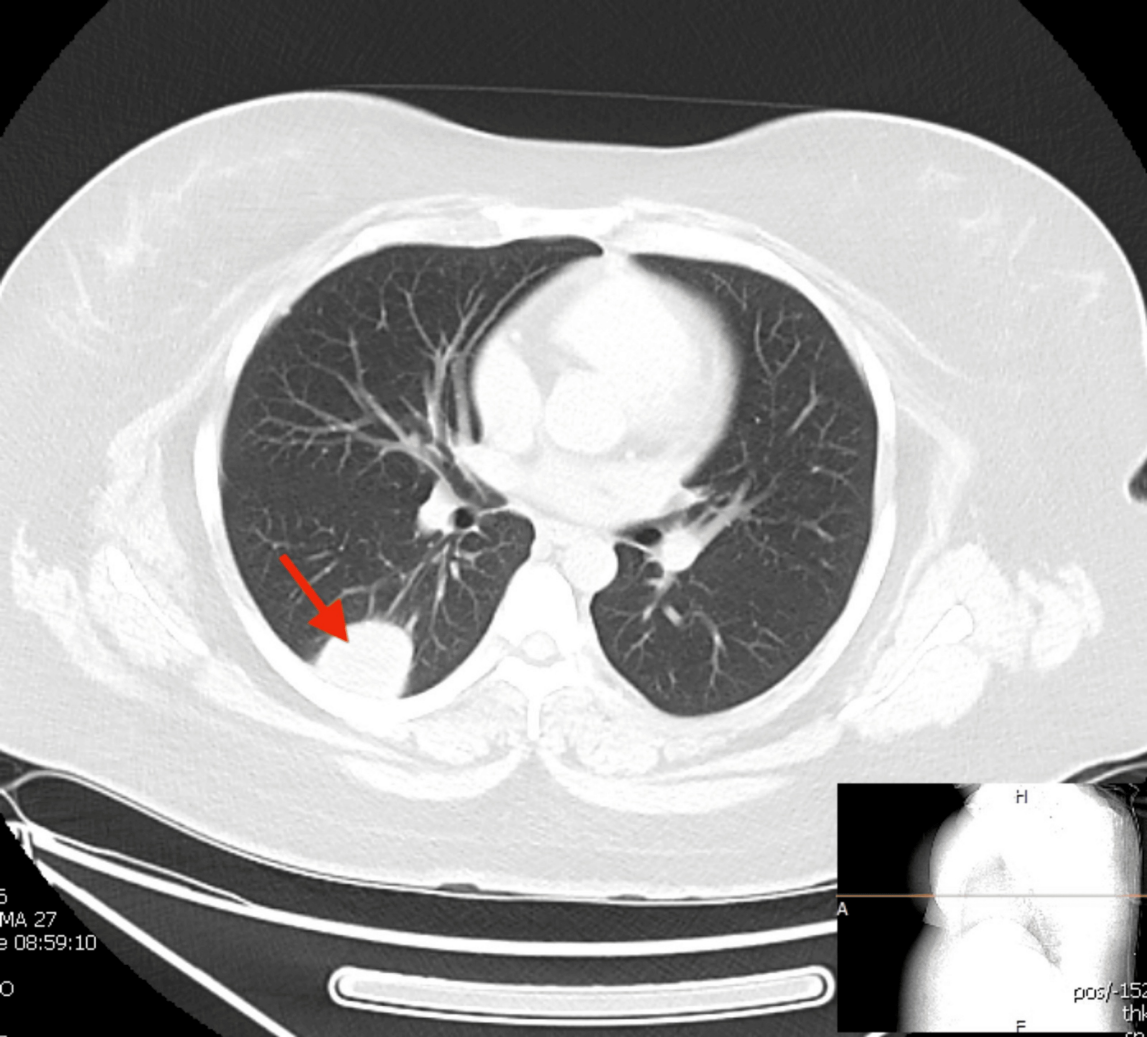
Computed tomography scan of the chest showing a pulmonary nodule. Image authorized by the patient.
